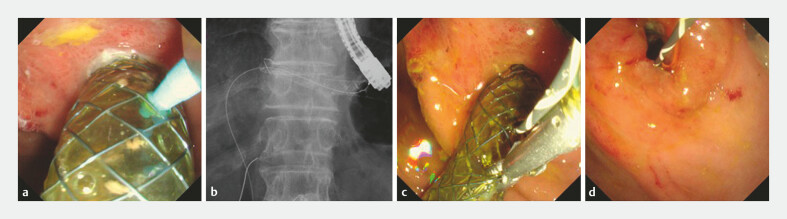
Technical tips for endoscopic ultrasound-guided hepaticogastrostomy (EUS-HGS) stent removal using the through mesh technique.
